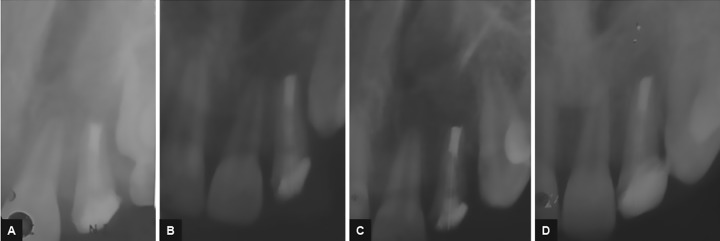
Intraoral periapical radiographs: (A) First year; (B) second year; (C) third year; and (D) fourth year follow-up
